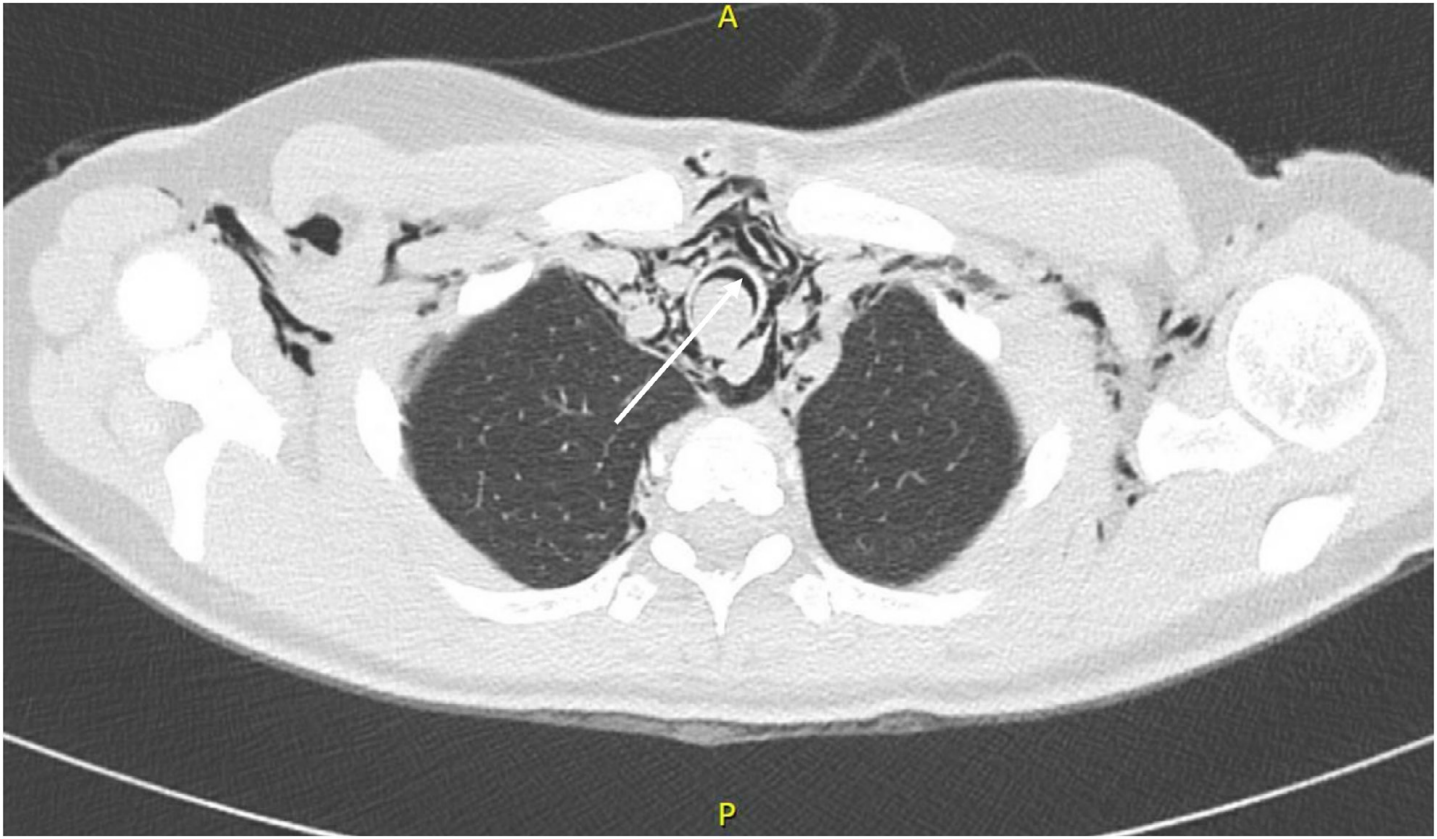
Chest computed tomography (CT) scan. White arrow shows near total obstruction of the tracheal lumen by the tracheal schwannoma.
